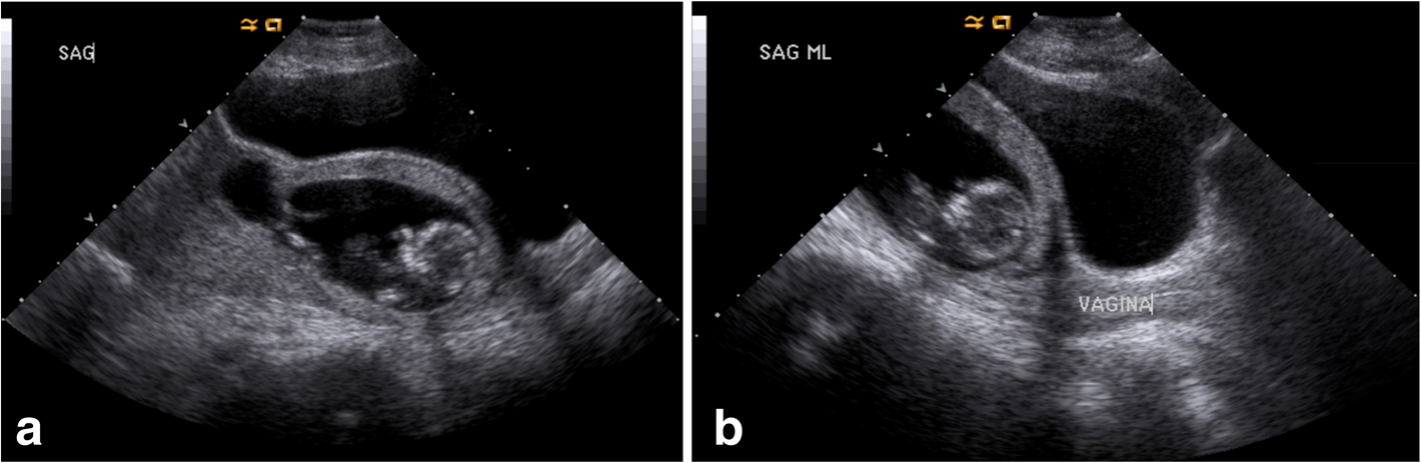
Sagittal transvaginal ultrasounds demonstrating cesarean scar ectopic pregnancy located in close proximity to bladder (anteriorly). Image on the left shows a very thin myometrium (2-5 mm) between gestational sac and bladder
